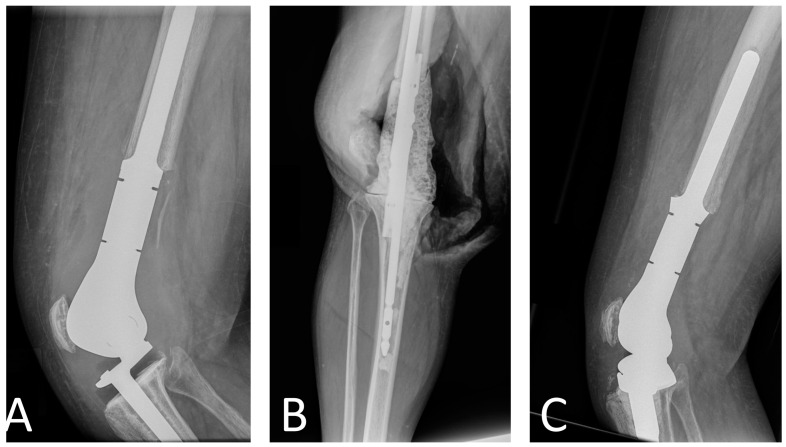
Radiographs of patient with distal femur replacement PJI treated with two-stage revision. (A) Pre-explantation radiographs, (B) intraoperative radiographs of the spacer with two tibial nails, and (C) post-reimplantation radiographs.
